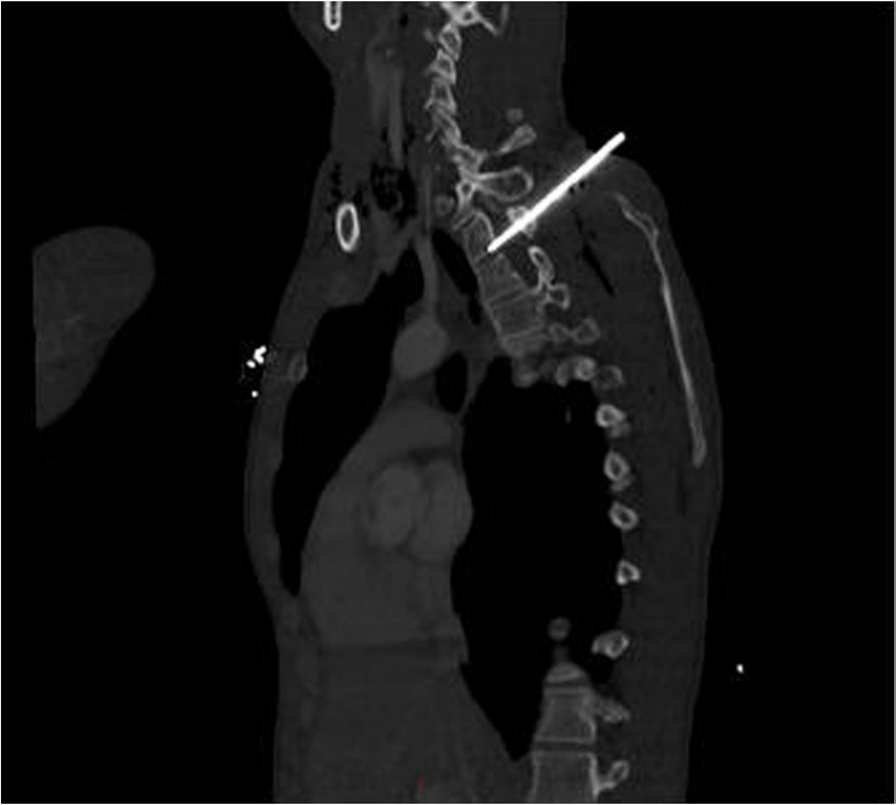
Sagittal CT of thorax demonstrating position of the knife corresponding the T2 and T3 spinal level
